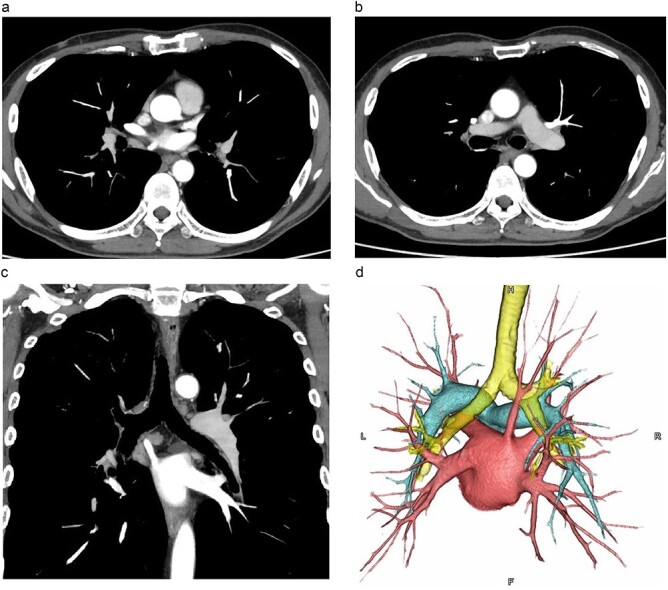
CT images. (a, b: axial images, c: coronal image, d: 3D image). The vessel was observed arising from the PVs draining the posterior upper lobe, running posterior to the RMB, and draining into the LA.
